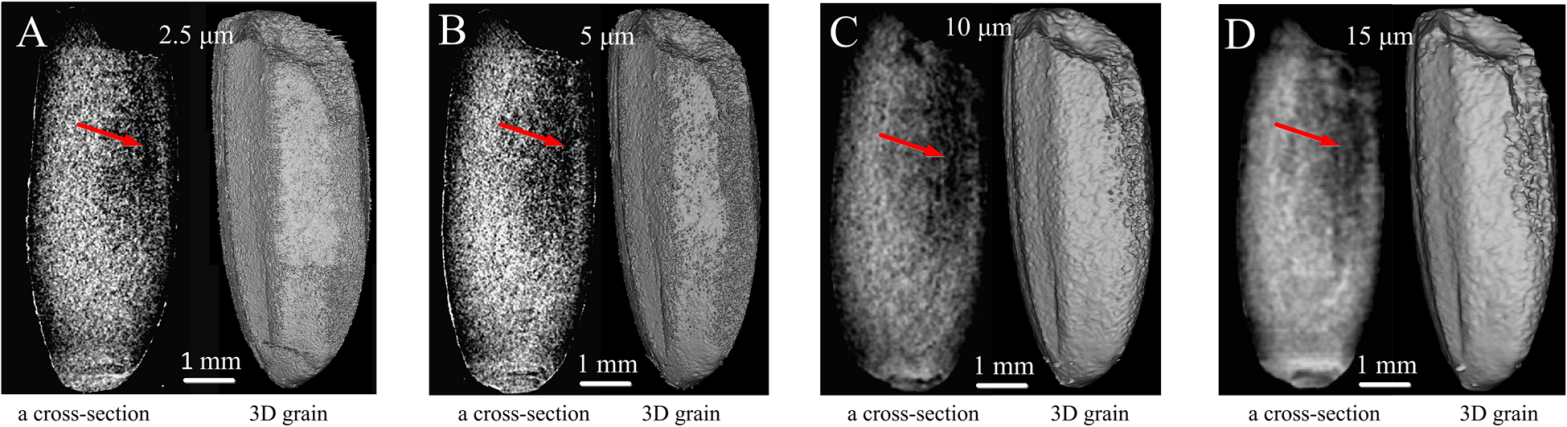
3D reconstruction of rice (X220) under different X-ray scanning resolutions. A cross-section image and reconstructed 3D milled rice kernel with whit-belly chalkiness under X-ray scanning with resolution of 2.5 μm (a), 5 μm (b), 10 μm (c) and 15 μm (d). Dark areas indicated by red arrow represented the location of rice chalkiness
